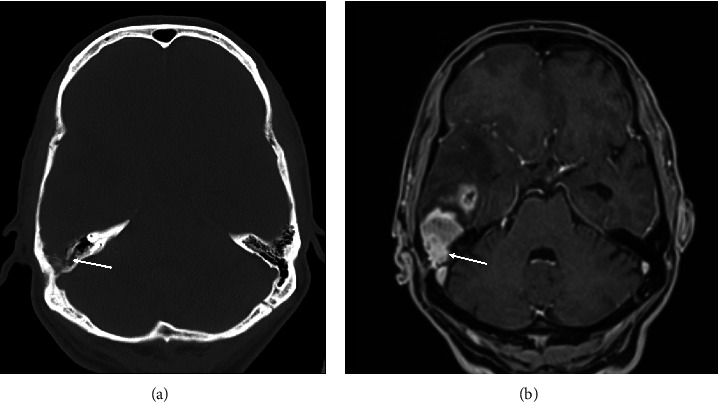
Axial plain CT (a) and contrast-enhanced MRI (b) at the level of the petrous ridges. (a) CT visualizes the osseous destruction of the right petrous ridge (arrow). (b) Contrast-enhanced T1-weighted MRI discloses infiltration of the GBM into the mastoid cells of the right temporal bone (arrow).
